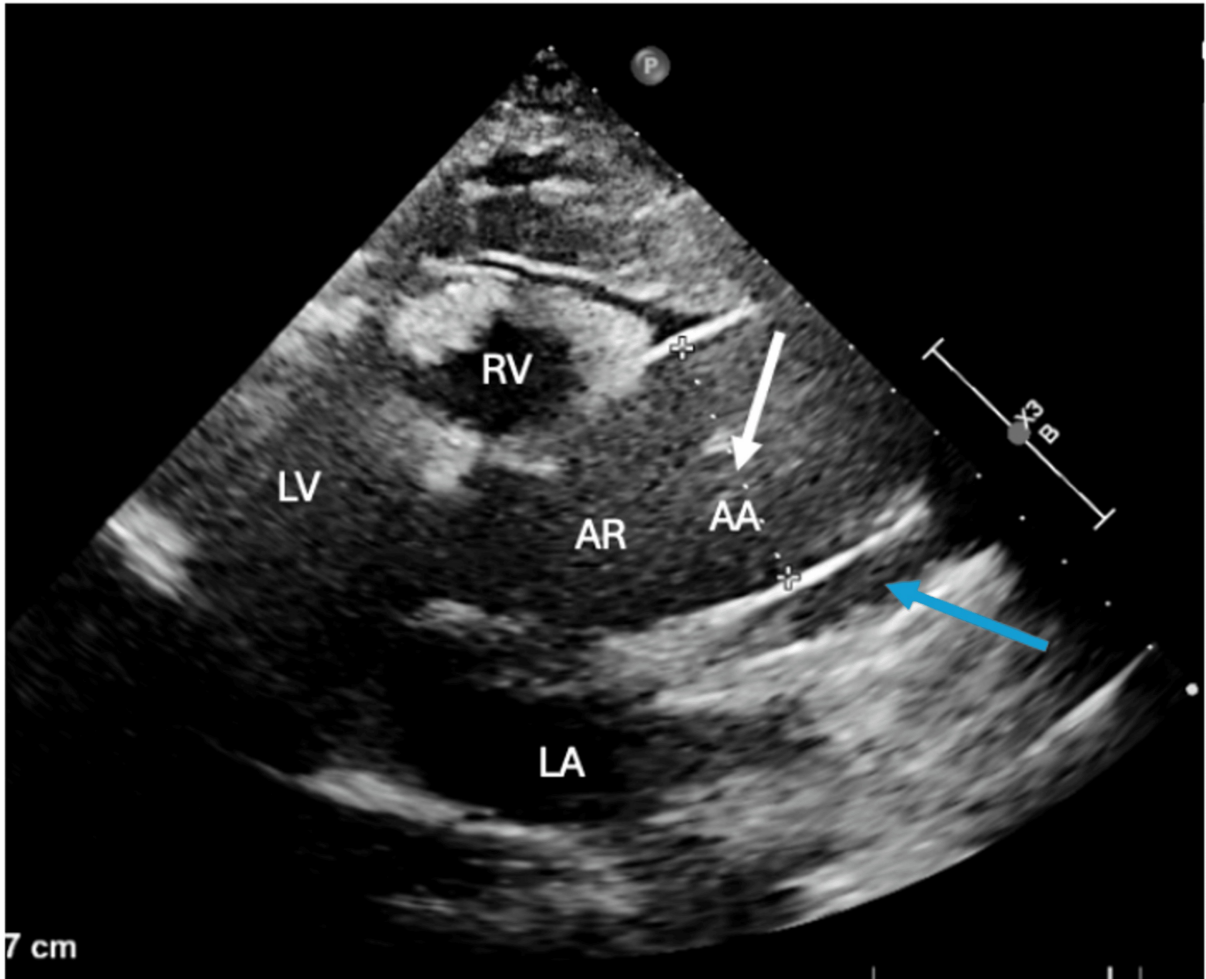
TTE in the parasternal long axis view exhibiting the Stanford type A aortic dissection, with dilatation of the ascending aorta (measuring 4.5 cm). The white arrow points to the true lumen, and blue arrow points to the false lumen. LA: left atrium; LV: left ventricle; RV: right ventricle; AR: aortic root; AA: ascending aorta; TTE: transthoracic echocardiography
